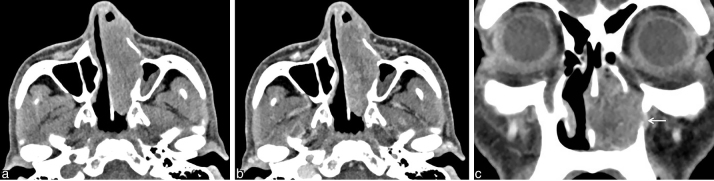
a–c. CT scan shows a mass occupying the left nasal cavity. (a) Axial noncontrast CT scan shows the iso-attenuating mass compared with muscle and no calcification. (b) Axial contrast-enhanced CT scan shows the mass is heterogeneously enhancing. (c) Coronal contrast-enhanced CT scan shows the mass occupying the left nasal cavity. The lateral wall of the maxillary sinus is osteolysed.
